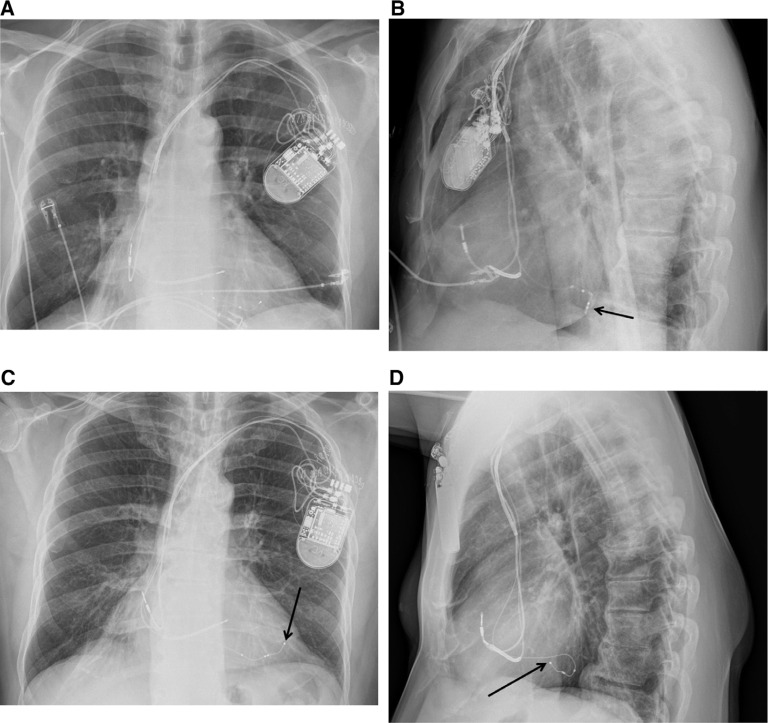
Posteroanterior (A) and lateral (B) chest X-rays demonstrating the position of the quartet lead. The posteroanterior (C) and lateral (D) chest X-rays reveal that the canted lead was able to reach a slightly different and more stable position. The arrows indicate the approximate position of the LV lead tip (distal electrode).
